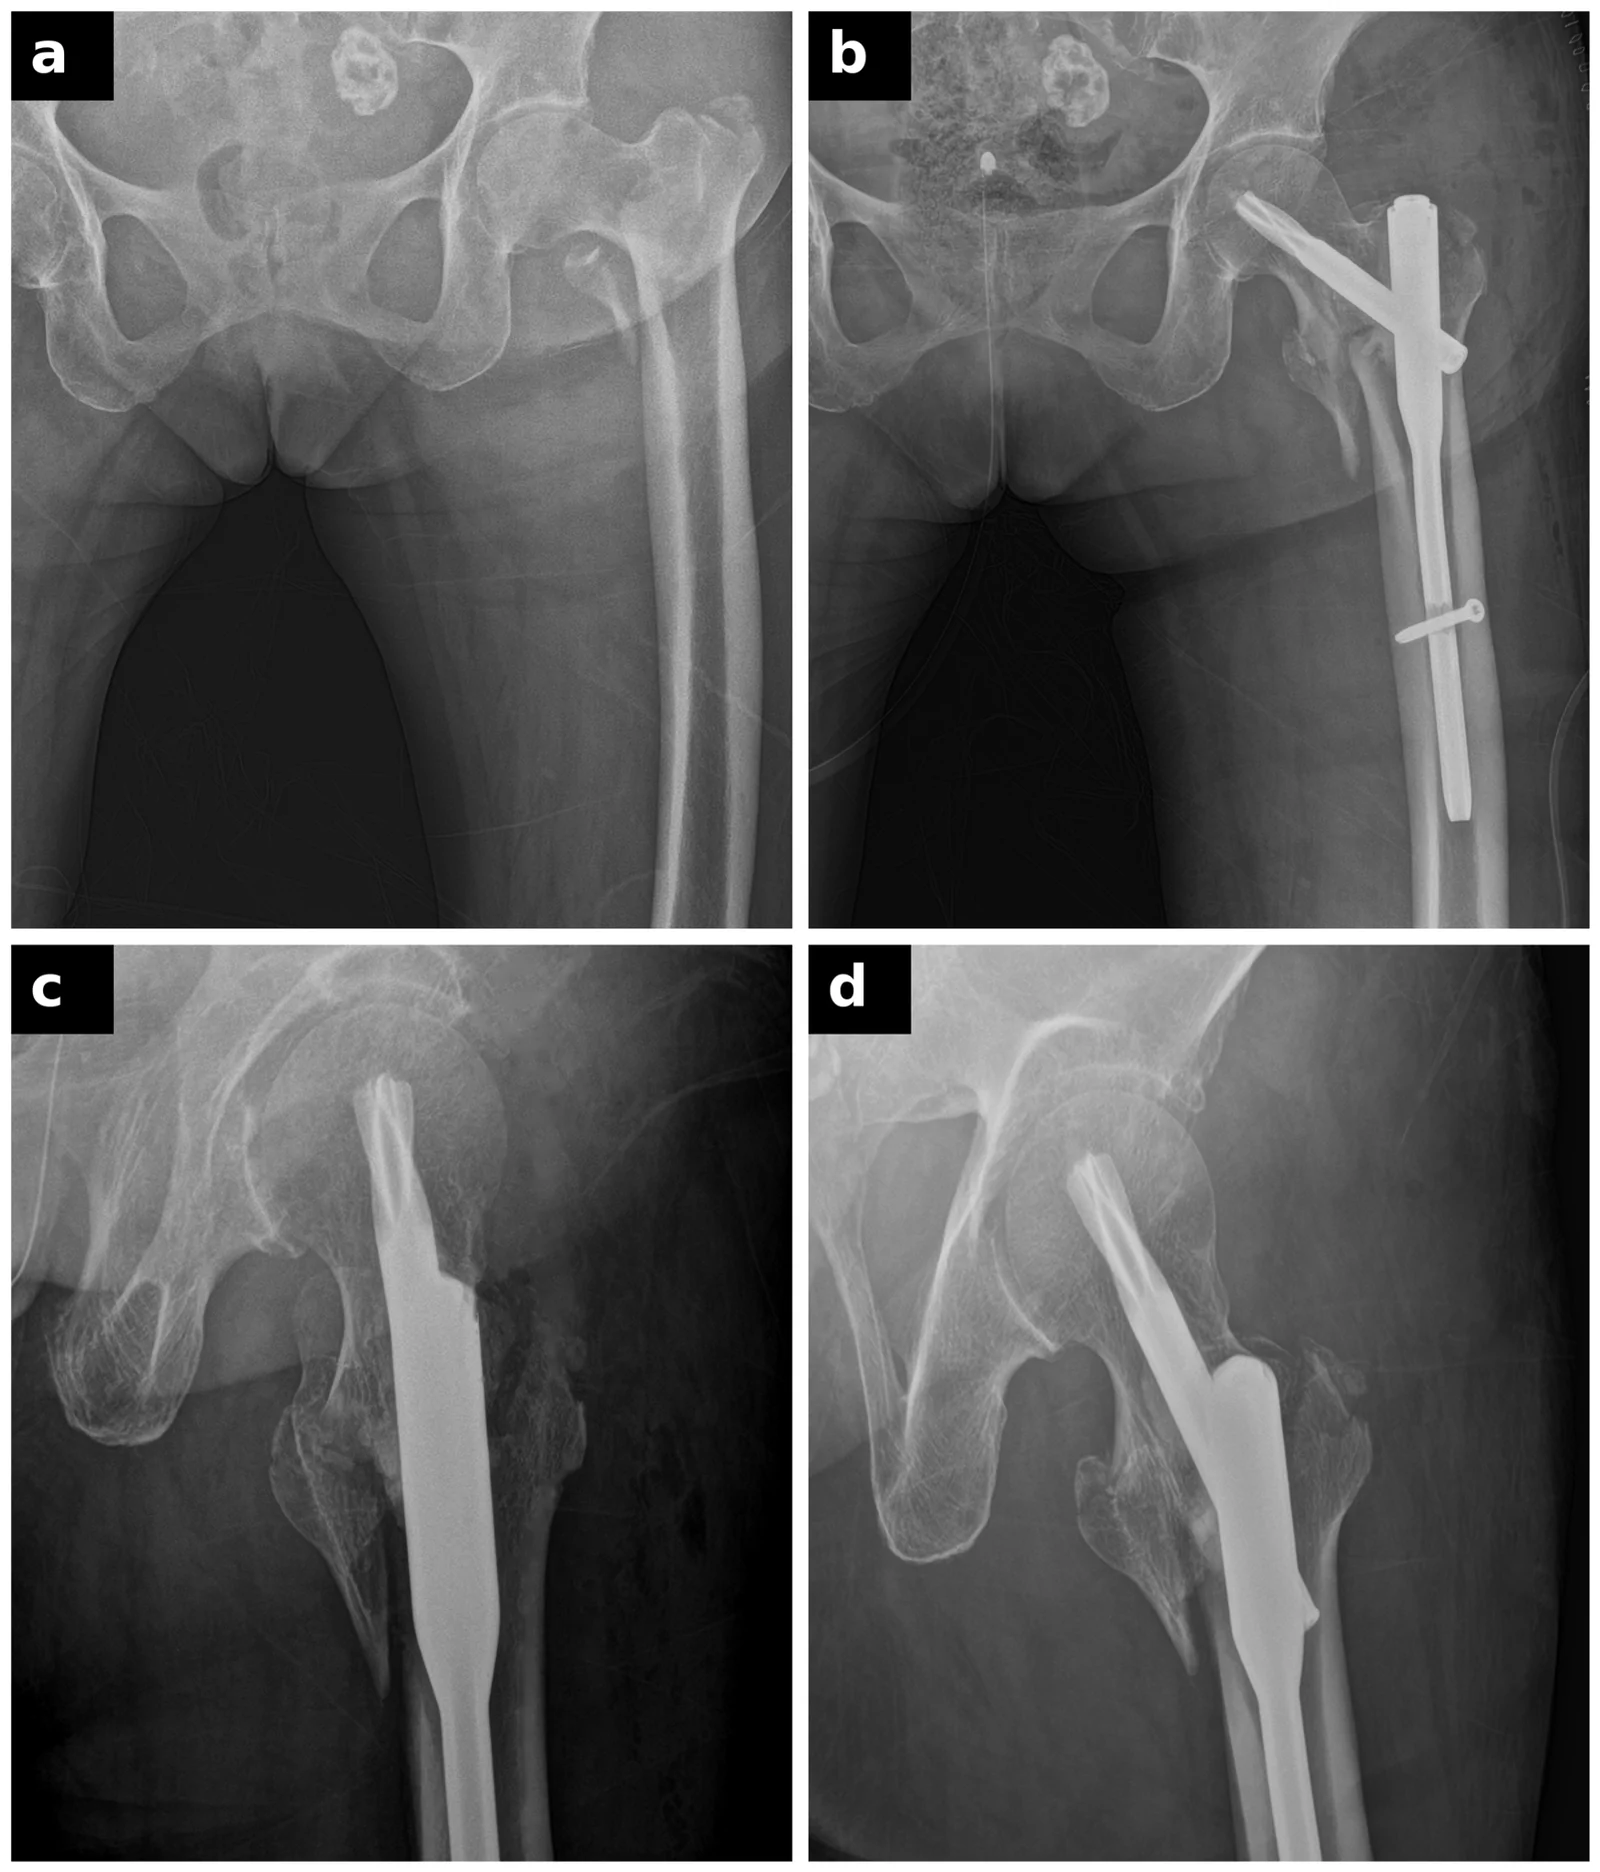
A 78-year-old woman with an AO/OTA 31-A2 fracture with a big lesser trochanteric fragment treated with a standard-length cephalomedullary nail. (a) Preoperative anteroposterior radiograph. (b) Immediate postoperative anteroposterior radiograph (day 0), showing the whole nail with one distal interlocking screw. (c) Immediate postoperative lateral radiograph (day 0), used as the baseline. (d) Lateral radiograph at the first outpatient visit (4 weeks after surgery): the proximal head–neck fragment has translated posteriorly around the nail with loss of anterior cortical contact (posterior sagging). Panels (a–d) are shown at the same magnification.
